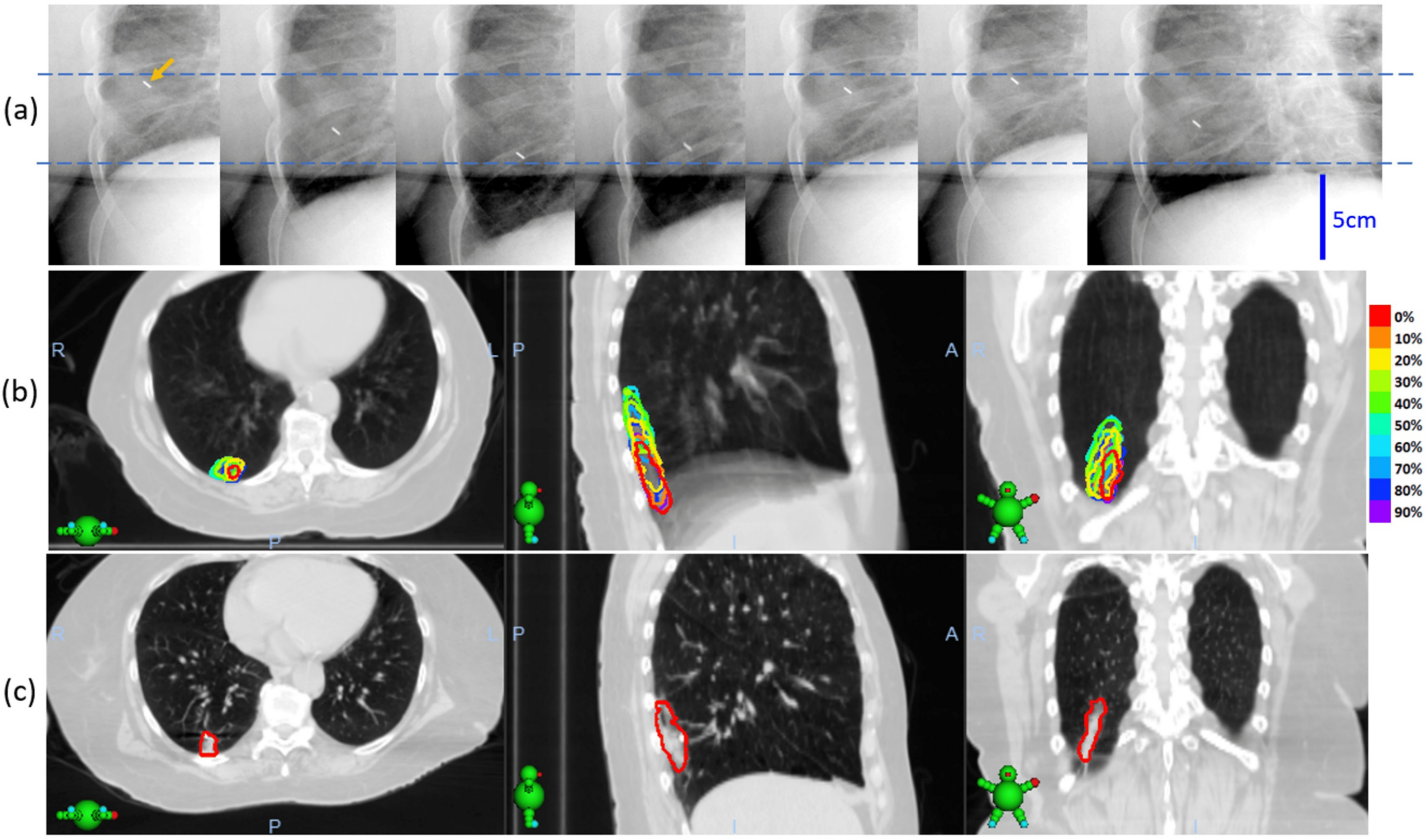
(a) Large breath-induced motion (~5 cm) of lung tumor observed on a series of kV images. The tumor has an implanted gold fiducial marker. (b) Tumor motion trajectory acquired using 4D-CT. The tumor was delineated separately for each respiratory phase, with each phase represented by a different color, as indicated in the color bar. (c) Real tumor shape and size delineated on breath-hold CT scans. 4D-CT: four-dimensional computed tomography, CT: computed tomography
